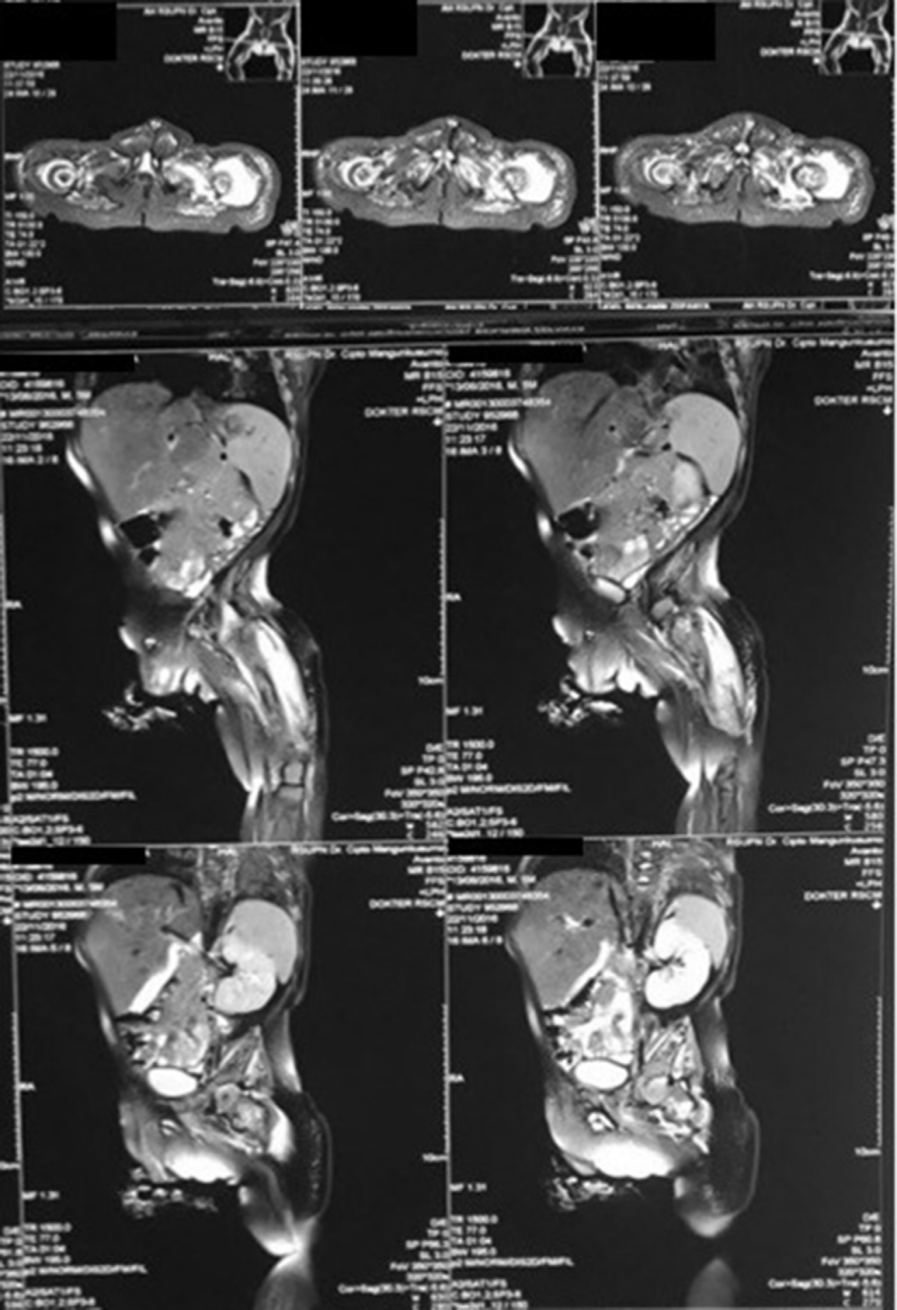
First MRI of pelvic and left thigh concluded multifocal osteomyelitis (2016).
